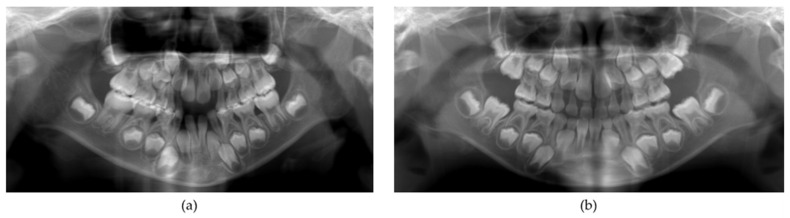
Panoramic radiography: (a) Older sister (seven years and three months); (b) Younger sister (six years and one month).
